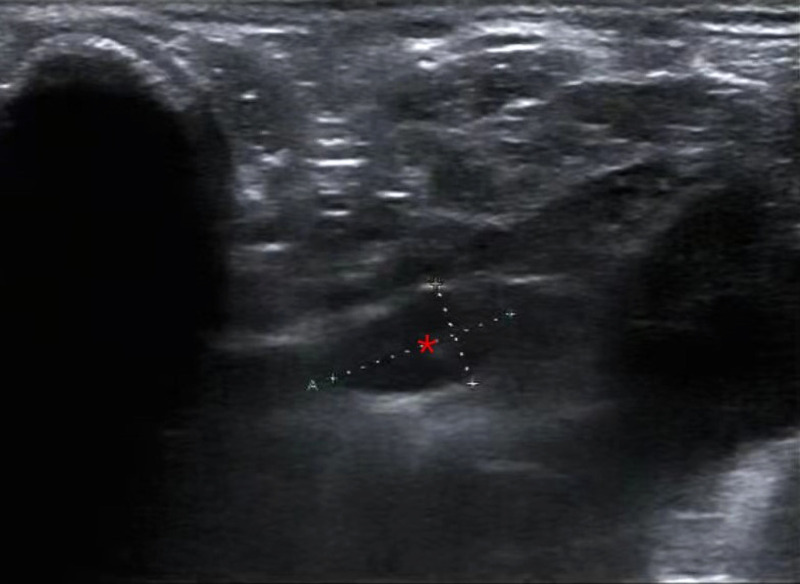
Parathyroid ultrasound showing a hypoechoic nodule (*) on the left side of the suprasternal fossa.
